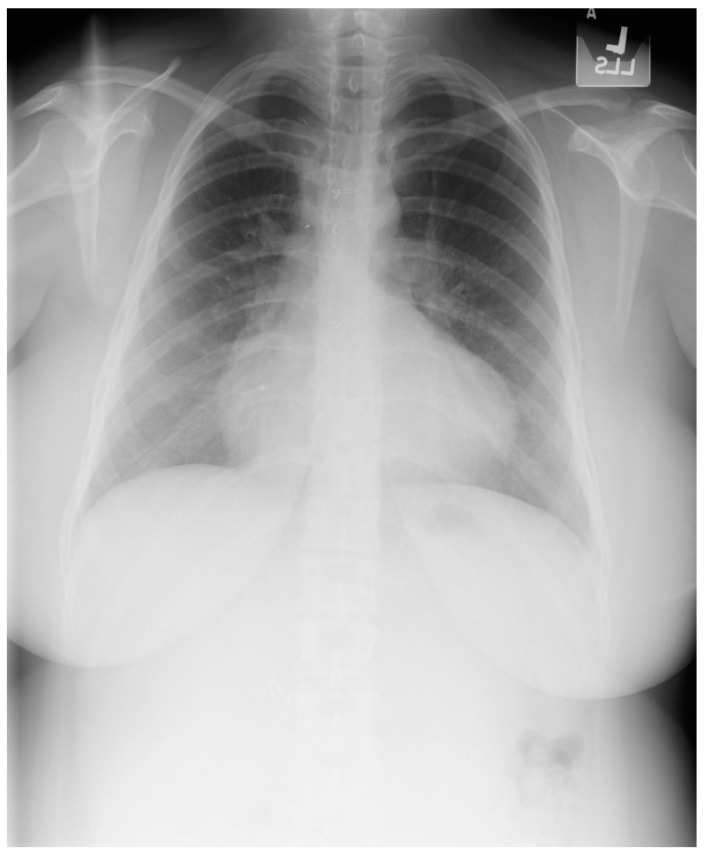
Posteroanterior chest X-ray in an adult with previously operated tetralogy of Fallot: the chest X-ray was obtained in a 21-year-old female with exertional dyspnea. She was cyanotic at birth and diagnosed with tetralogy of Fallot. She had a Blalock-Thomas-Taussig (BTT) shunt (palliative subclavian artery to pulmonary artery shunt) at 10-months-of-age. At 3-years-old she underwent complete repair with closure of the ventricular septal defect, right ventricular (RV) outflow tract reconstruction with pericardium, with transannular patch and ligation of the BTT shunt. This posteroanterior chest X-ray is well centered and the inspiration is good. The cardiothoracic ratio is increased and the cardiac silhouette is boot-shaped (“coeur en sabot”). The right heart border is prominent, suggesting right atrial enlargement. The pulmonary vascularity is normal and the lung fields and pleura are clear.
